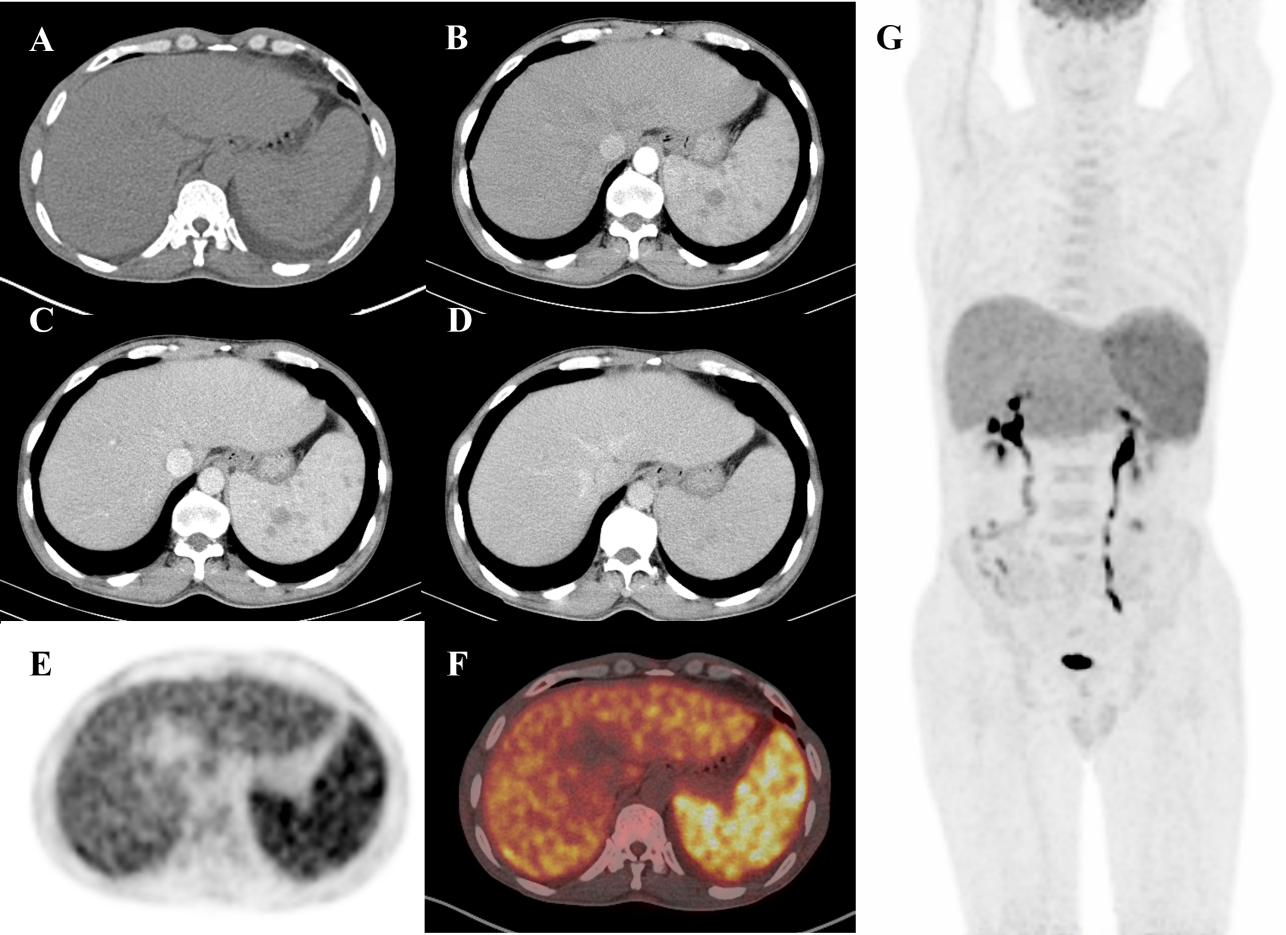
A 55-year-old man presented with a fever for 1 month. Routine diagnostic testing for fever of unknown origin (FUO) did not lead to the diagnosis of etiology. The patient was referred to the Department of Nuclear Medicine for an ^18^F-FDG PET/CT scan (axial CT, enhanced CT, PET, fusion, and maximum intensity projection (MIP)). Axial non-contrast CT (A) only revealed splenomegaly. However, PET (E) and fusion (F) images showed diffuse FDG uptake in the enlarged spleen with a maximum standardized uptake value (SUV max) of 6.6. The MIP image (G) displayed increased FDG uptake in the enlarged spleen, accompanied by multiple low-density nodules with delayed enhancement on abdominal enhanced CT (B–D), which were similar to those found in lymphoma. Based on pathological examination, a diagnosis of visceral leishmaniasis (VL) was made following splenectomy. The patient received treatment with amphotericin B and was discharged from hospital without fever.
